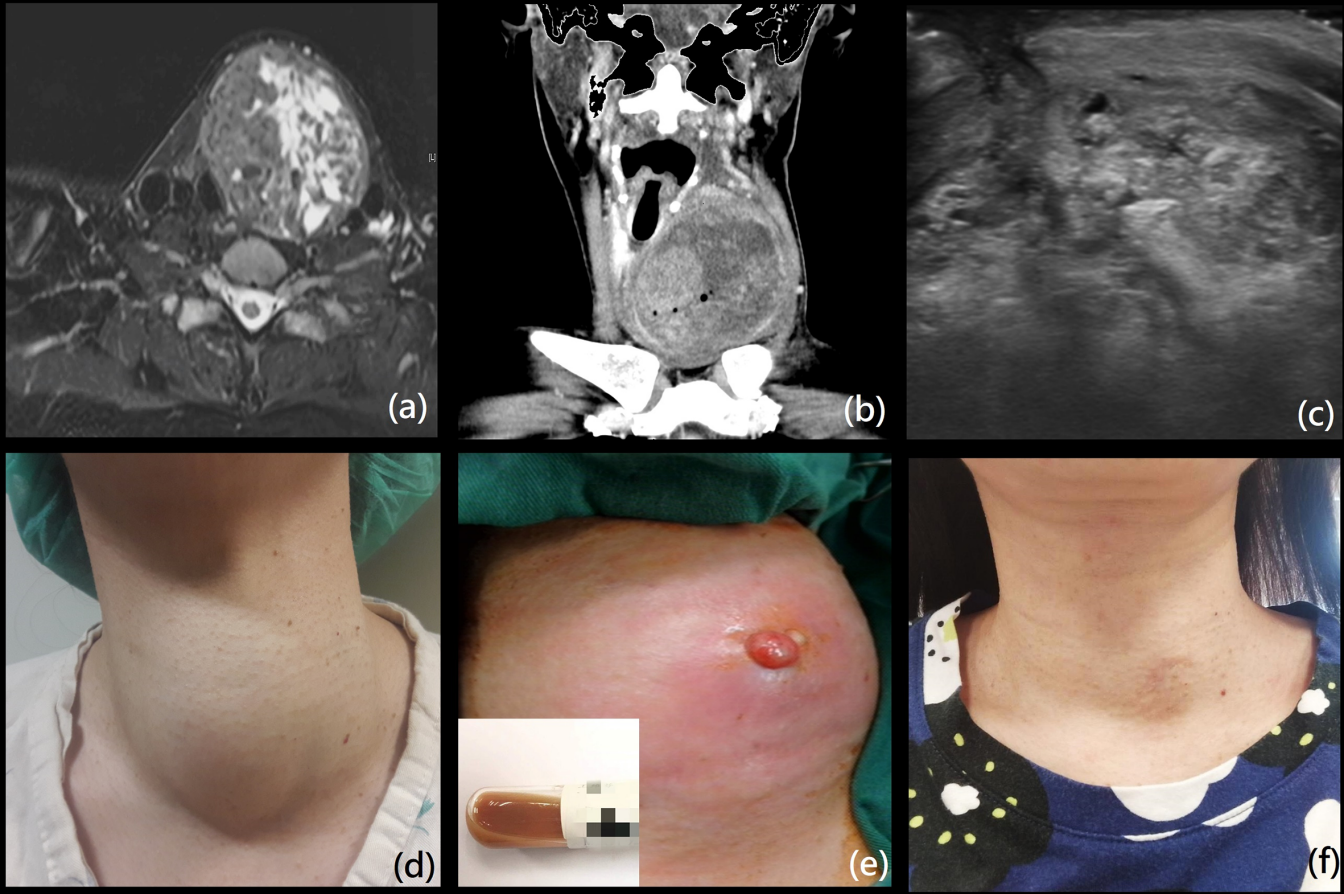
(A) A 44-year-old woman presented with a huge mixed-intensity thyroid mass, with initial size of 8.1 x 7.9 x 5.9 cm (volume 198ml) on MRI. She received one RFA session with total energy 25.14 kCal and procedure time of 50 min and 43 sec. (B) Sudden swelling and erythema on the left neck was noted 19 days after the RFA. Coronal view of computed tomography demonstrated necrosis of nodule with anterior capsule disruption with fluid extension subcutaneously (anterior type). The patient was admitted for intravenous antibiotics and surgical debridement; brown mucous content without hemorrhage were noted. She was hospitalized for 7 days. (C) The lesion gradually regressed to size of 4.5 x 2.9 x 3.7 cm at 1 month after surgery on echo. (D–F) The patient’s appearances on the time prior to RFA, at debridement, and two years after RFA. We have obtained the patient’s consent to apply her photographs and information in the article.
